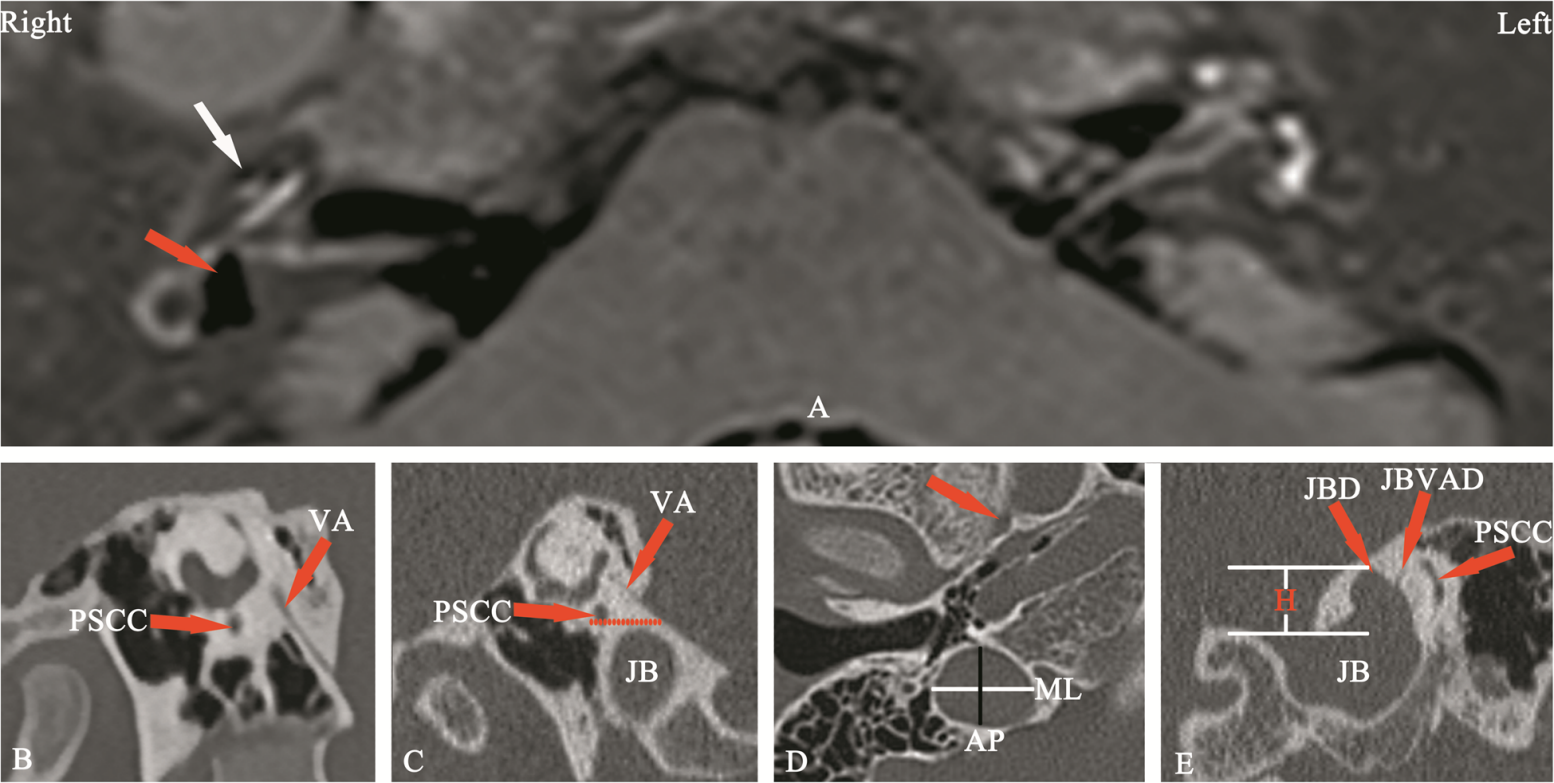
a 3D real inversion recovery sequence MRI showing a mild cochlear EH (white arrow) and a significant vestibular EH (red arrow) in the right ear and there is no pathological finding in the left ear. b The external aperture of the VA can be well visualized in the Pöschl plane. When failing to visualize this structure, non-visualization of the VA was recorded. In this image, JB was classified as type 1 because of no bulb. c The external aperture of the VA can also be well visualized in the Pöschl plane. In this image, JB was classified as type 2 because of the JB below the inferior margin of the PSCC. d Anteroposterior (black line) and mediolateral (white line) diameters of the JB were recorded on the axial image at the level where the foramen spinosum (arrow) could be observed. e The height of the JB was recorded by measuring the distance between the level of the JB dome and the line passing through the confluence of the sigmoid sinus with the JB on the coronal image. The JBVAD was defined as when the bony coverage separating the JB and VA is dehiscent. The JBD was defined as when a prominent protrusion or outpouching of the JB can be clearly distinguished from a smooth ellipsoidal form on the coronal image. EH, endolymphatic hydrops; JB, jugular bulb; JBD, JB diverticulum; JBVAD, JB-vestibular aqueduct dehiscence; H, height; AP, anteroposterior; ML, mediolateral; PSCC, posterior semicircular canal; VA, vestibular aqueduct
